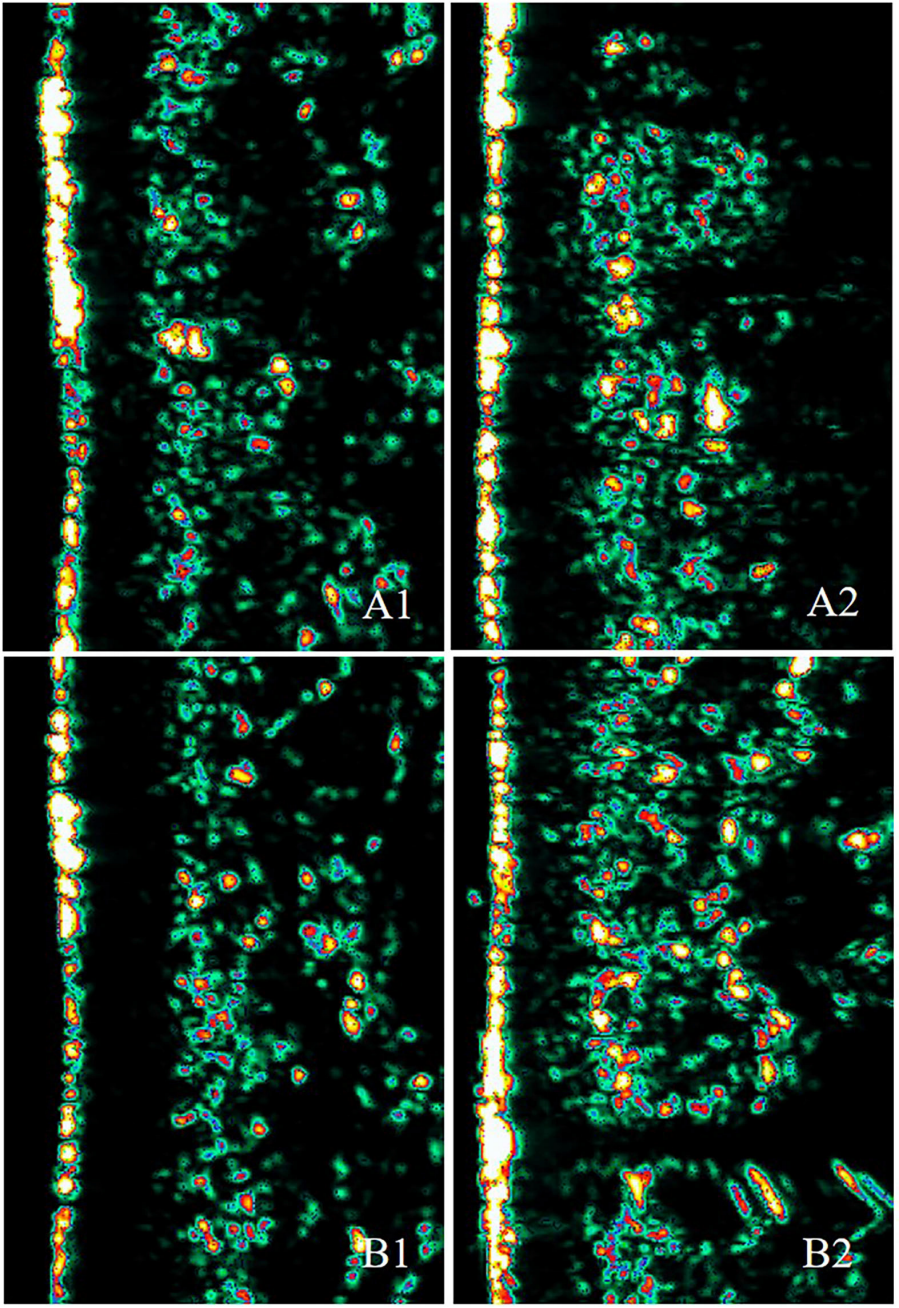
Ultrasound images of a 40-year-old woman at the baseline and at 2 weeks after the last treatment. A1 and B1 show images taken at the baseline (A1: skin elasticity = 5.3, skin thickness = 1,290.3 μm; B1: skin elasticity = 5.3, skin thickness = 1,158.7 μm). A2 shows the image taken 2 weeks after the last ECM-C *via* MN treatment (skin elasticity = 5.6, skin thickness = 1,422 μm). B2 represents the image taken 2 weeks after the last ECM-C *via* MN and RF treatment (skin elasticity = 6.1, skin thickness = 1,457 μm). Echogenicity color scale: white>yellow>red>green>black.
